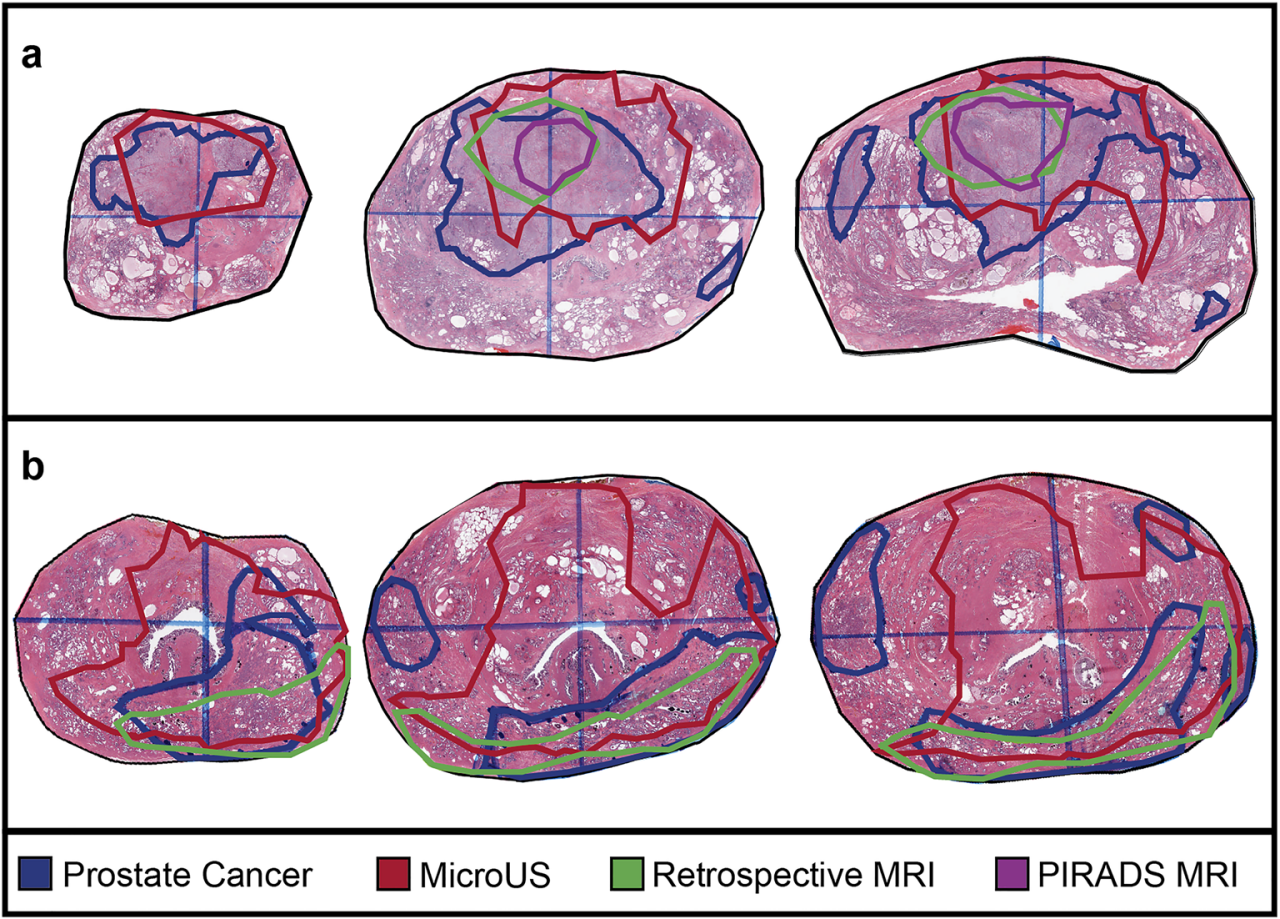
Example co-registration outputs of microUS and MRI with WM pathology for two cases (a and b).
